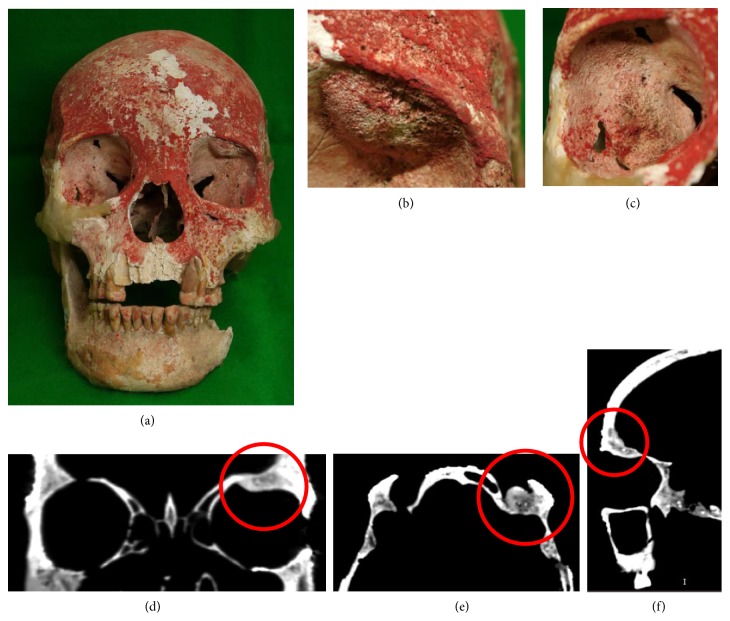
Photographs of the skull. Red pigment was applied mainly on the frontal face region after death (a). In the left superior orbital wall, a 15 × 15 mm bulging lesion with a slightly porous surface was present (b) in which a solid osteoblastic lesion continuous with the inner region was noted on CT images (d, e, and f). On the inner wall surface of the right orbit, a 20 × 20 mm irregular prominent lesion was present (c).
